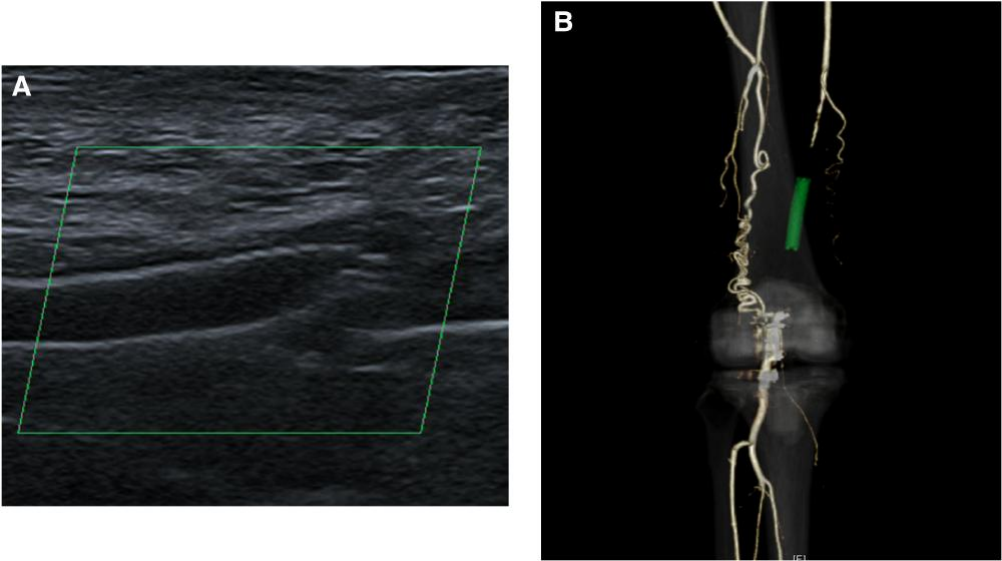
Imaging studies six months after the third endovascular treatment. (*A*) Ultrasonography demonstrated popliteal artery occlusion secondary to Supera stent fracture. (*B*) Contrast-enhanced computed tomography scan showed occlusion from the superficial femoral artery to the popliteal artery despite the development of collateral blood channels.
